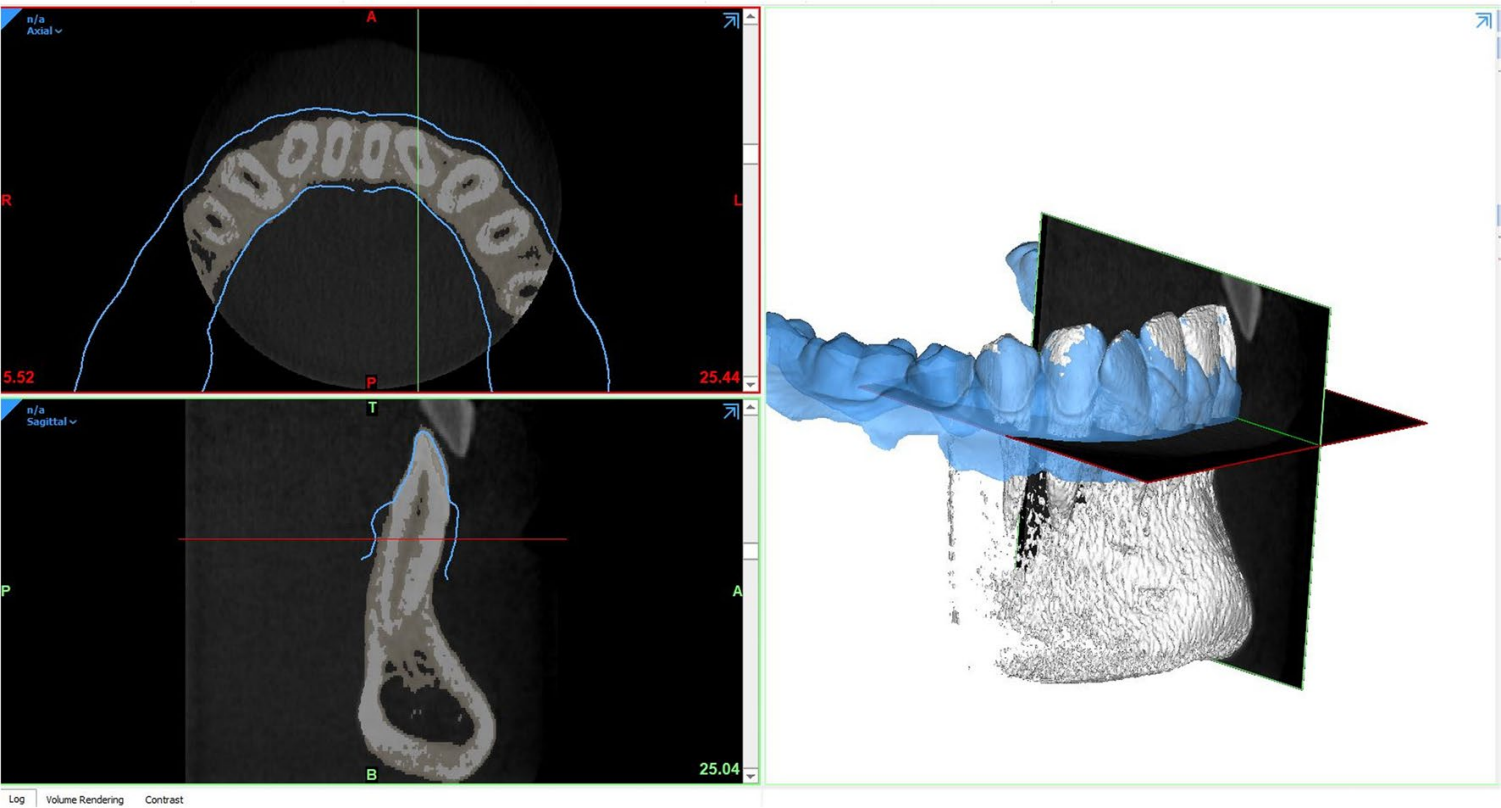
Representative example of the superimposition of an intraoral scan (blue color) with the CBCT dataset in Materialize Mimics after the creation of a 3D reconstruction from the DICOM data
